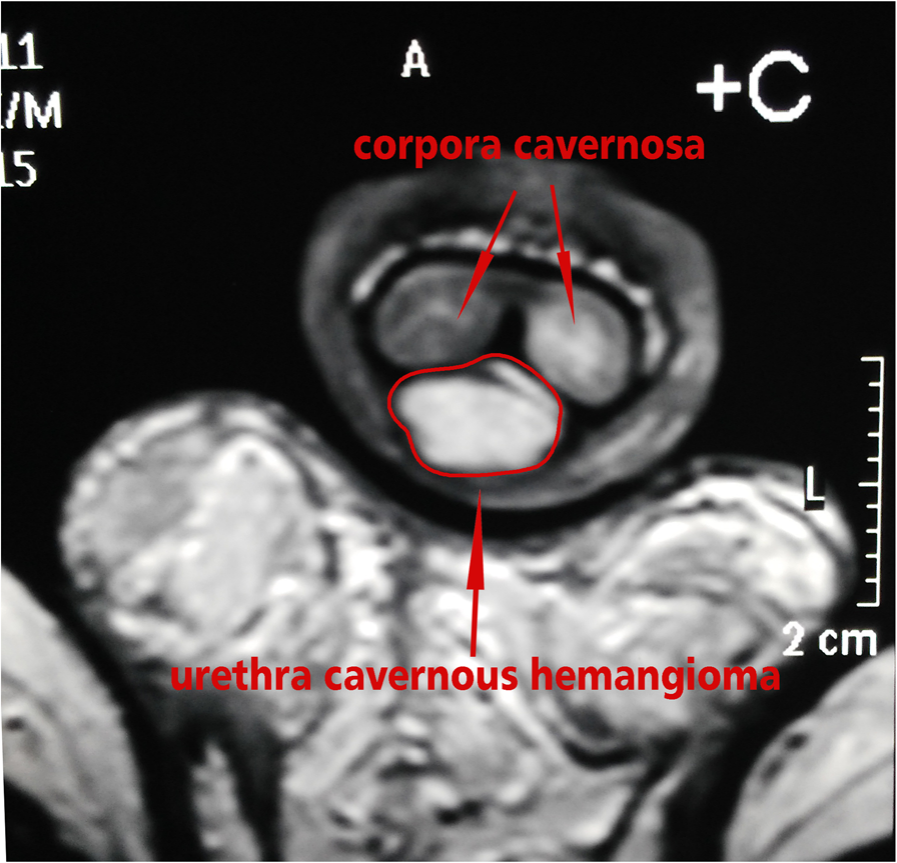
Preoperative penis coronal MRI shows a high signal on the ventral side of the penis, and underneath the urethra cavernous bodies of the penis
